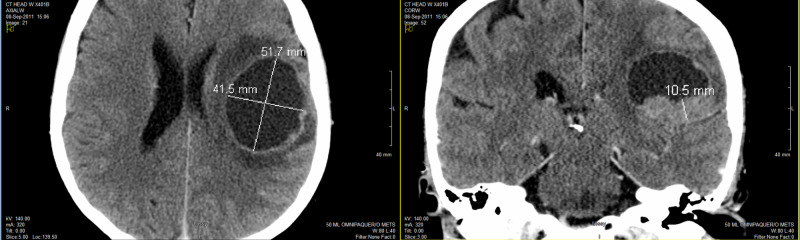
CT of the head showing a large 5.2-cm mass in left parietal brain causing extensive vasogenic edema and midline shift to the right side
